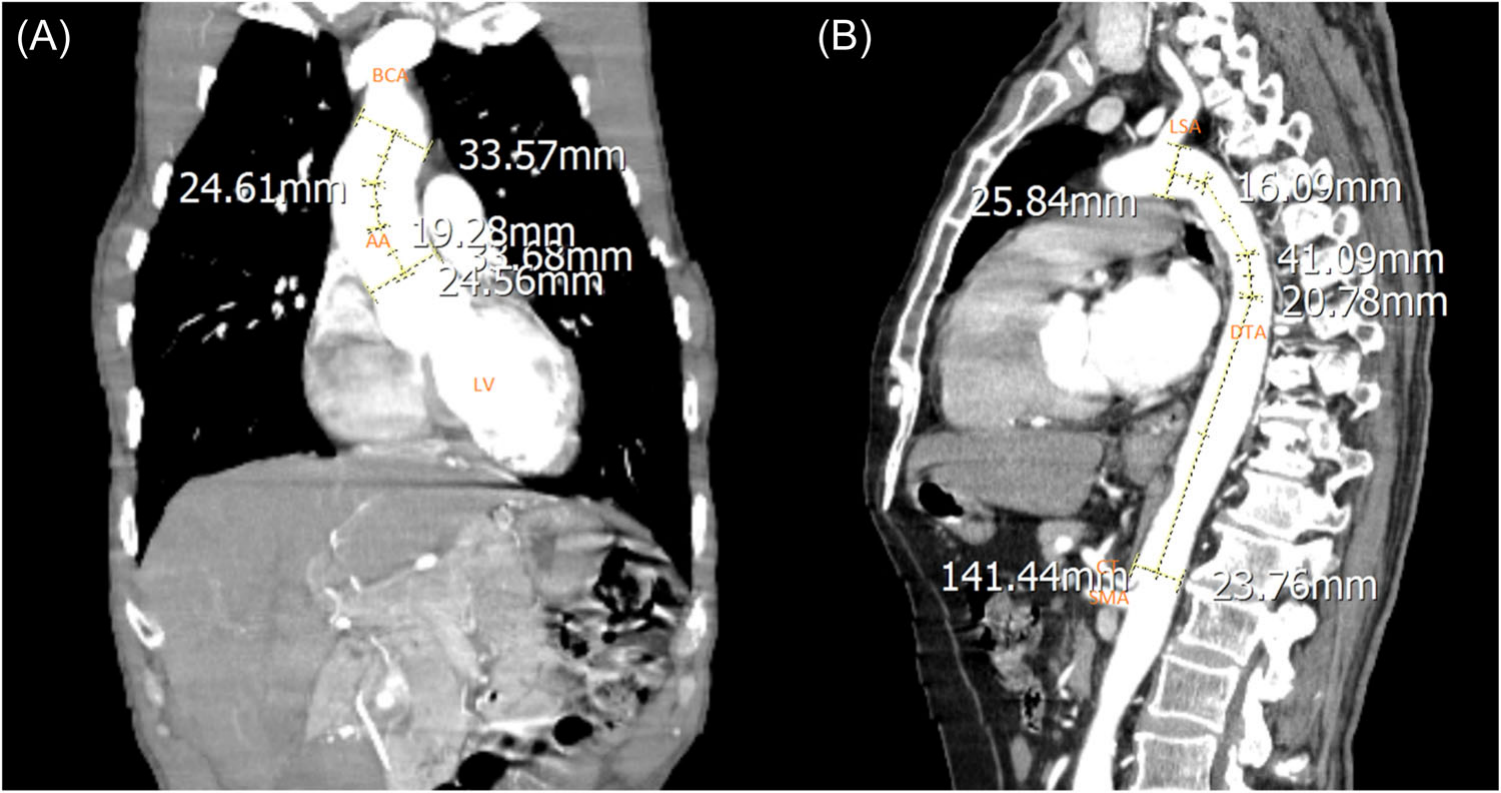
Computed tomography scan with Coronal (A) and Sagittal (B) view, demonstrating applied measurements in our study. (A) The length of the ascending aorta from the sinotubular junction to the brachiocephalic detachment and the diameter of the ascending aorta at the proximal and distal end; descending (B) The length of the descending aorta and the diameter of the descending aorta at the proximal and distal end (AA: Ascending aorta; DTA: descending thoracic aorta; BCA: brachiocephalic artery; LCC: left common carotid artery; LSA: left subclavian artery; LV: left ventricle; PA: pulmonary artery; LPA: left pulmonary artery; RPA: right pulmonary artery; CA: celiac artery; SMA: superior mesenteric artery).
